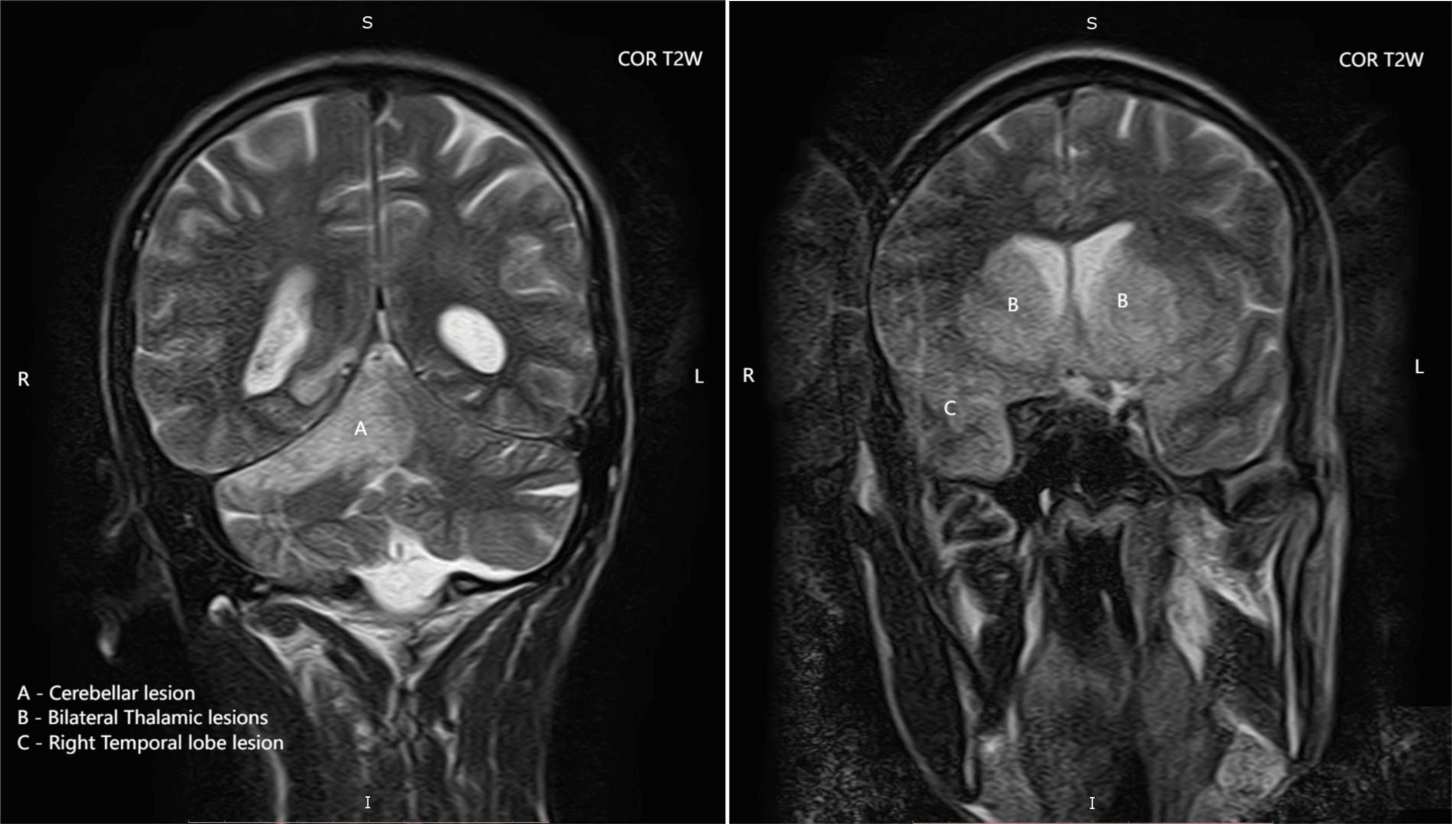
Coronal T2-weighted MRI demonstrating high signal intensity lesions involving (A) the cerebellum, (B) the bilateral thalami, and (C) the right temporal lobe MRI, Magnetic resonance imaging
